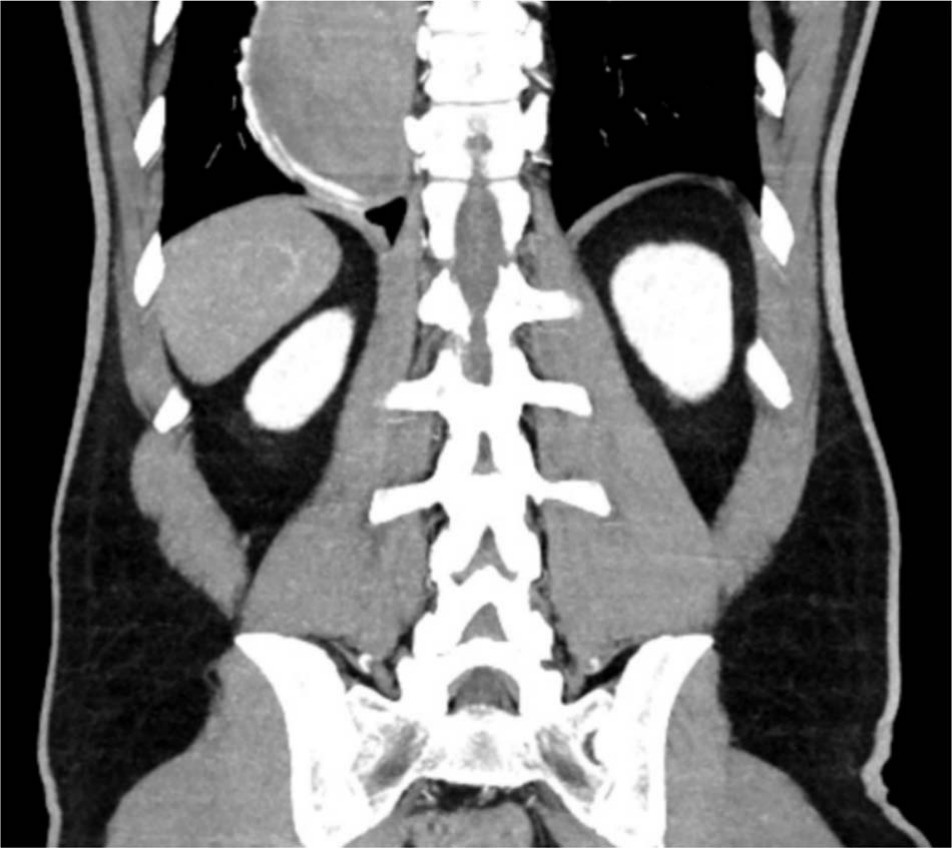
CT scan showing a markedly dilated esophagus with contrast blush in the distal third.
